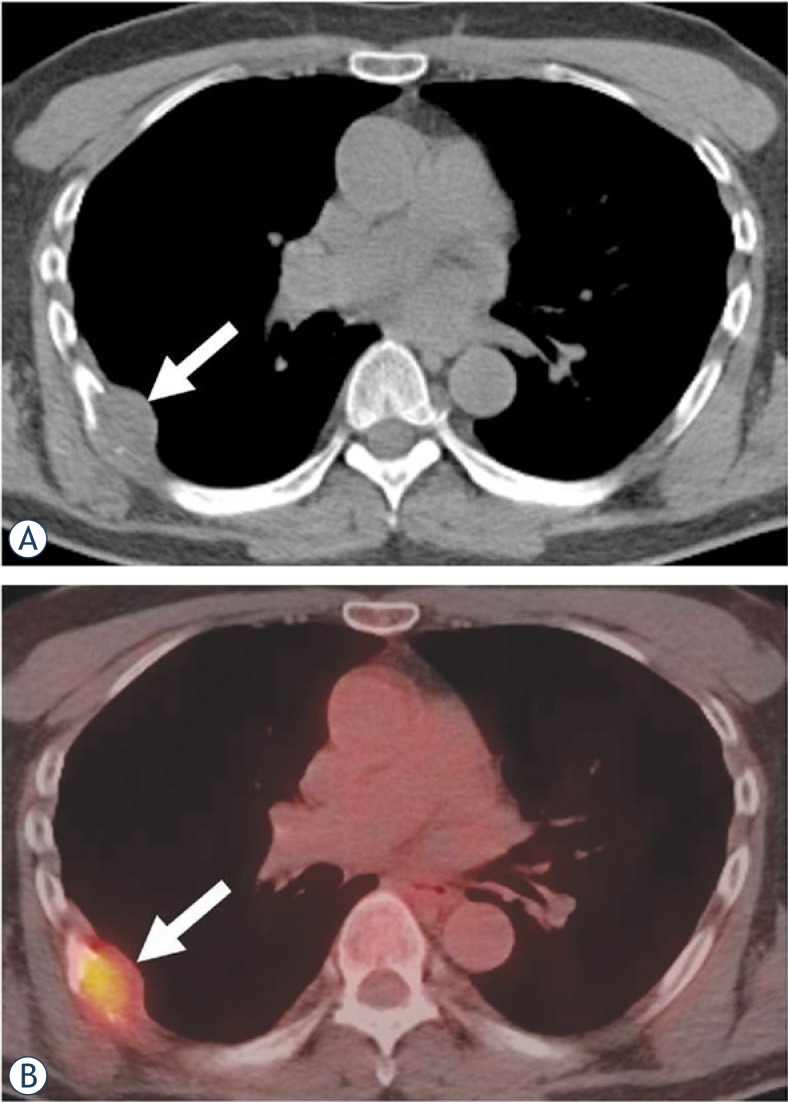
A 60-year-old man with left chest wall MPNST. A. Axial unenhanced CT image shows a low attenuation right chest wall mass abutting a rib (arrow) causing rib destruction and adjacent pleural thickening. B. Axial fused PET/CT image shows presence of moderate fluorodeoxyglucose (FDG) uptake (arrow, standardized uptake value [SUV]_max_ 4.4).
